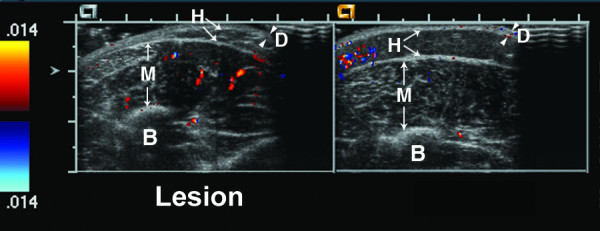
6 year old girl with linear scleroderma of her calf for the past two years. The lesion is warm and mildly violaceous. There is loss of hypodermal fat in the lesion (left side of lesion image). The lesion muscle layer has increased vascularity with at least 4 more large color Doppler signals on the lesion side compared to the normal muscle (deep tissue vascularity = 3). The Doppler signal seen on the left hand side in the normal hypodermis layer is artifact. B = bone, D = dermis, H = hypodermis, and M = muscle. Arrowheads indicate boundaries of dermis, while arrows indicate boundaries of hypodermis and muscle.
